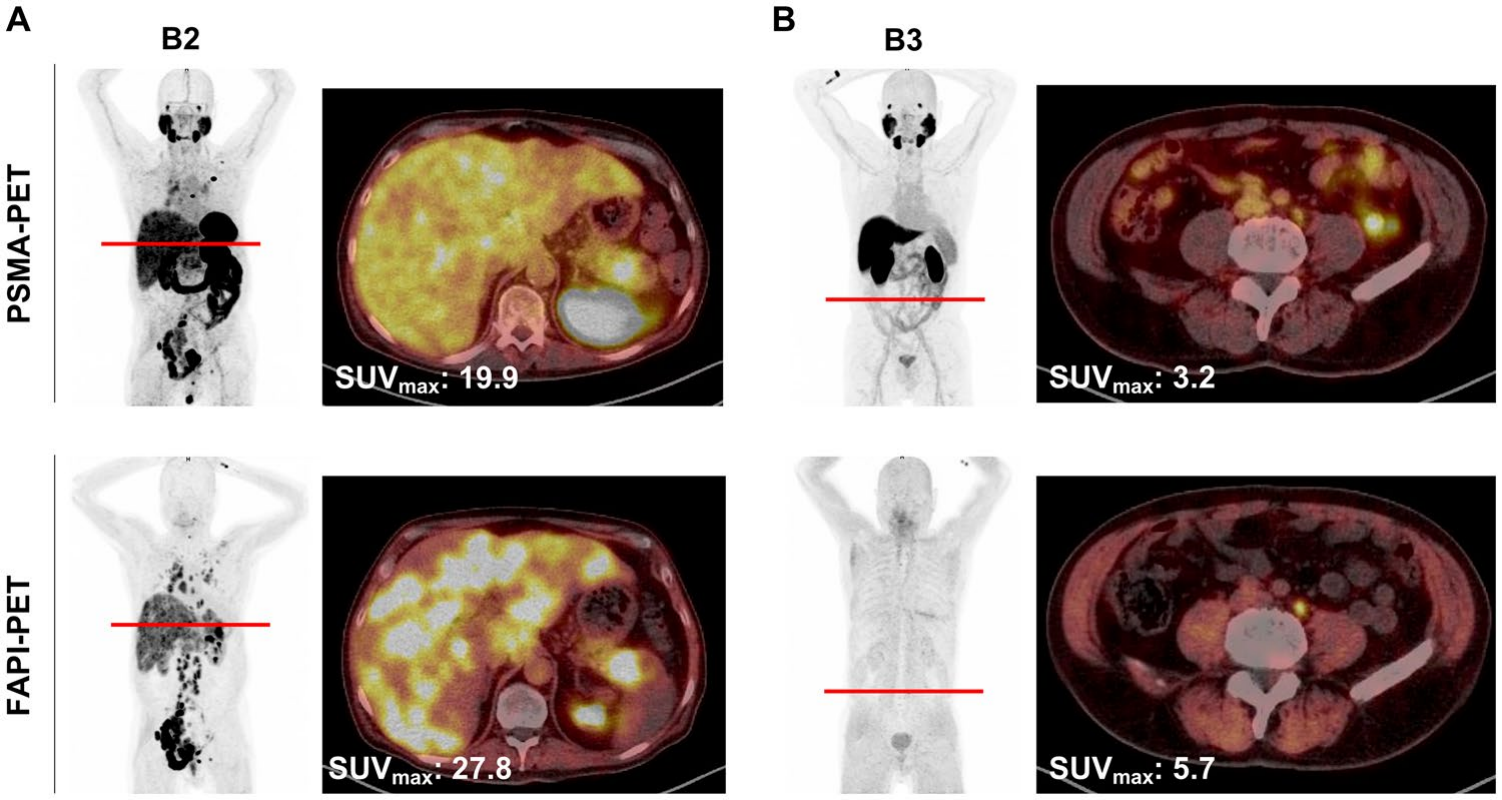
Whole body scans and axial images of cohort B patients. Patients with the highest (B2) and lowest (B3) SUVmax in PSMA scans were selected. Whole body scan and axial images are shown. Red lines indicated selected axis level. White numbers indicate SUV_max_ of the respective scan. *PSMA* prostate-specific membrane antigen, *FAPI* fibroblast activation protein inhibitor, *SUV*_*max*_ standard uptake value maximum, *PET* positron emission topography
